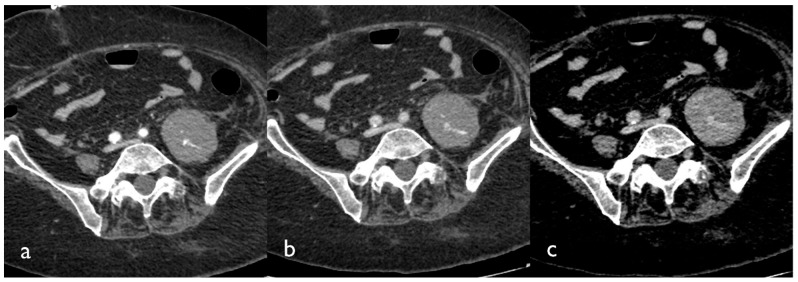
CT images of a patient presenting with a large hematoma of the left iliopsoas muscle. Tri-phase contrast-enhanced axial CT images show contrast blush in the arterial (a), portal (b), and delayed phase (c); active bleeding is seen like small hyperdense foci within the hematoma that appear in the arterial phase and grow in the venous and delayed phase.
